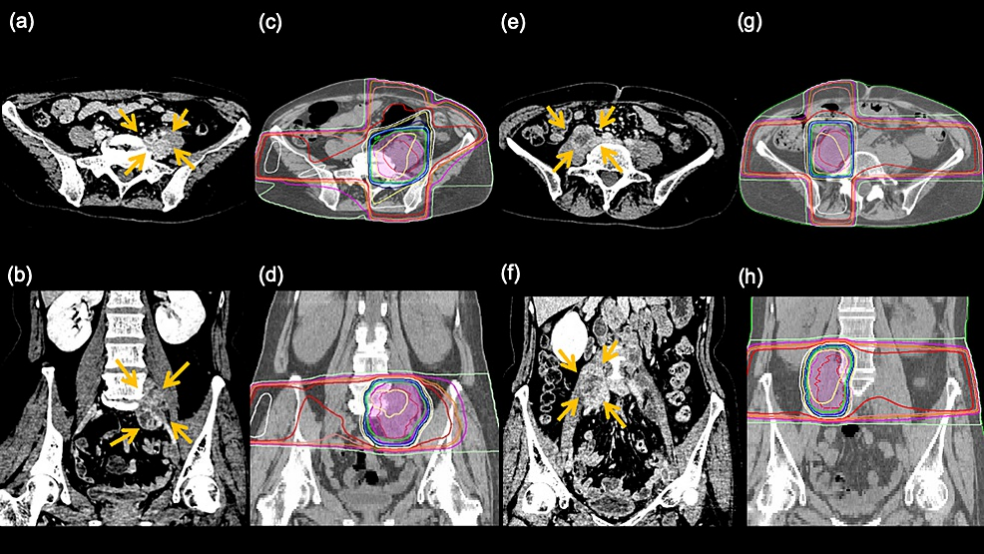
a 49-year-old woman with recurrent ovarian cancer (patient 1) The patient presents with bilateral involvement of the iliopsoas muscles. Palliative radiation is first administered to the left-sided tumor. (a) and (b): axial and coronal views of the tumor (arrows) on CT before irradiation. (c) and (d): radiation dose distribution with 95% isodose lines shown in green. Two weeks later, another course of radiation is performed for the right-sided tumor (e) to (h).
